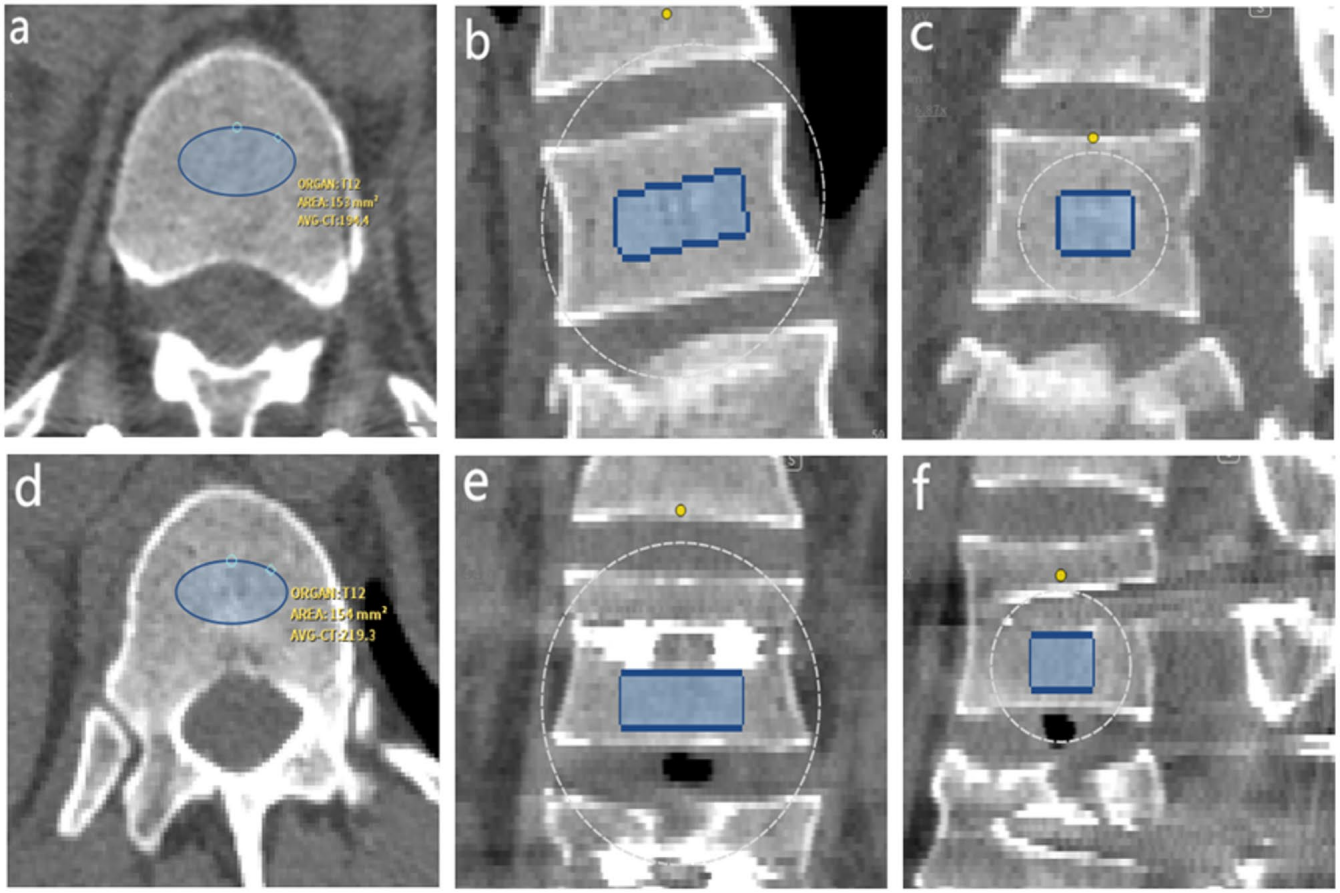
CT images showing bone density measurement assessments of the preoperative and postoperative vertebral body with pedicle screws in axial, coronal, and sagittal planes. Figures (a–c) present the axial, coronal, and sagittal measurements of bone density in the preoperative vertebral body, while Figures (d–f) display the axial, coronal, and sagittal measurements of bone density in the postoperative vertebral body.
